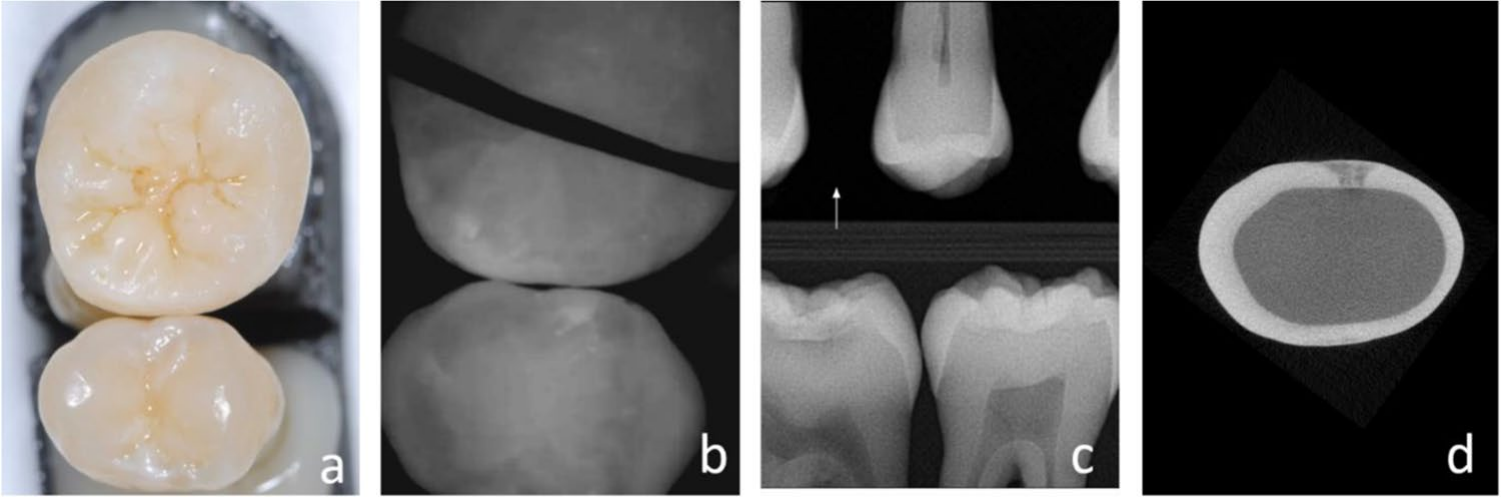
A non-cavitated caries lesion in a premolar that is visually undetectable (*a*). The caries lesion is visible with NIRR (white spot) and the black line marks the tooth that is not in the region of interest (*b*). The lesion was undetectable using X-rays and the arrow marks the side of interest (*c*). Micro-computed tomography reveals the presence of an initial dentin lesion (*d*)
